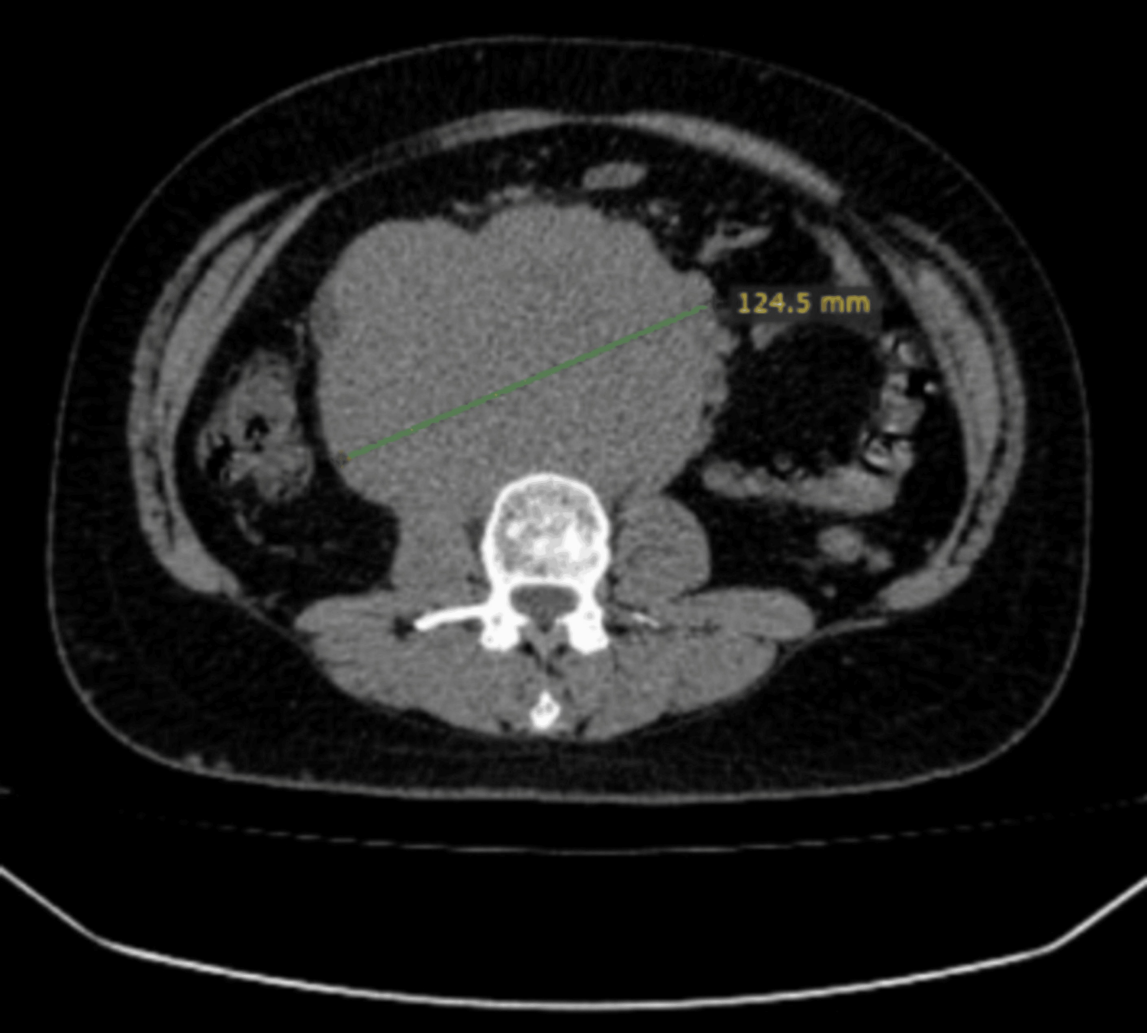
Axial image of a CT scan showing a mass of 11 cm in the abdomen CT: computed tomography
